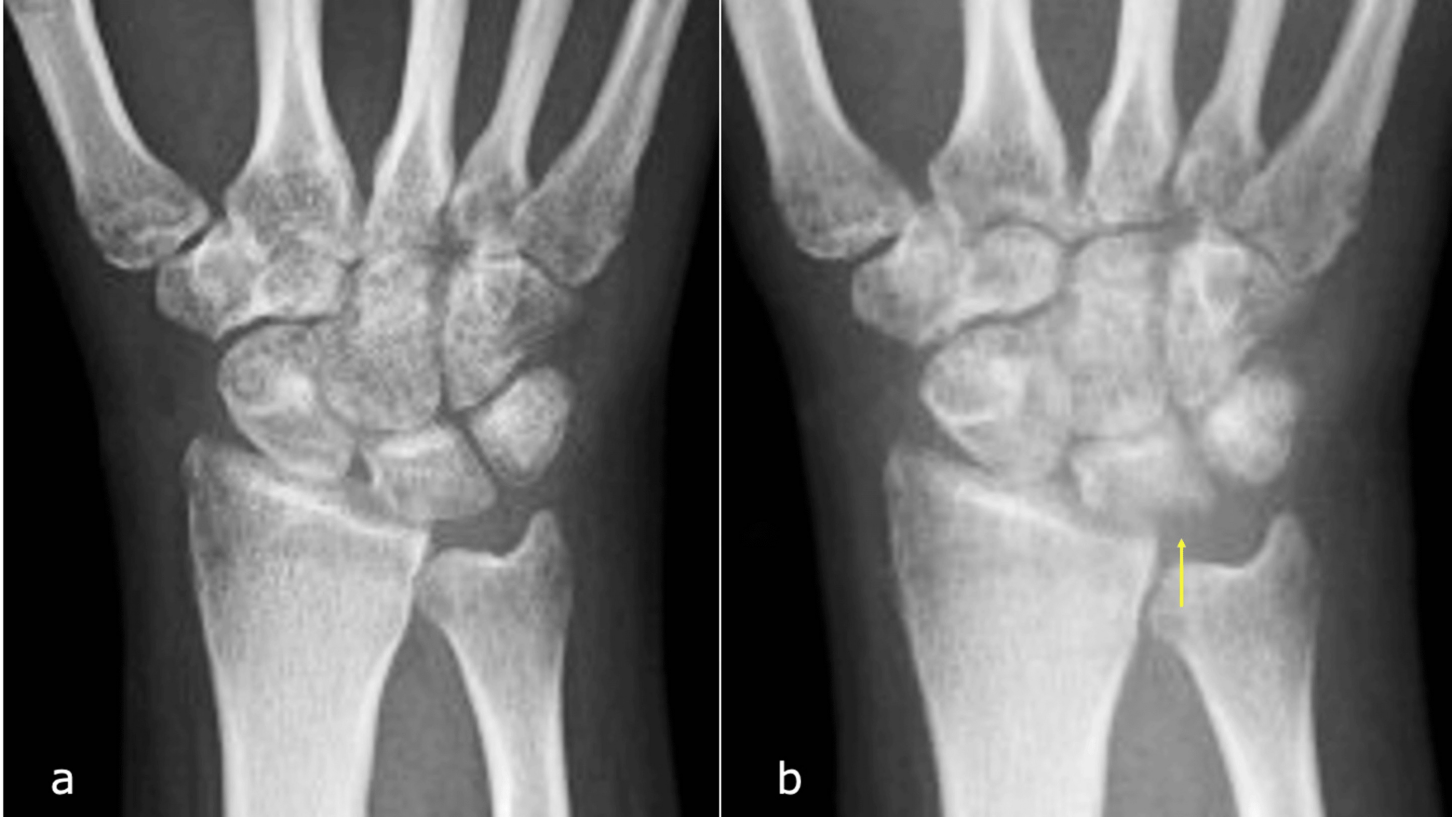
X-ray following Kirschner wire (K-wire) removal. a: Anteroposterior (AP) view immediately after K-wire removal. b: AP view three weeks post-K-wire removal. The irregularities of the proximal lunate articular surface can be seen (arrow).
